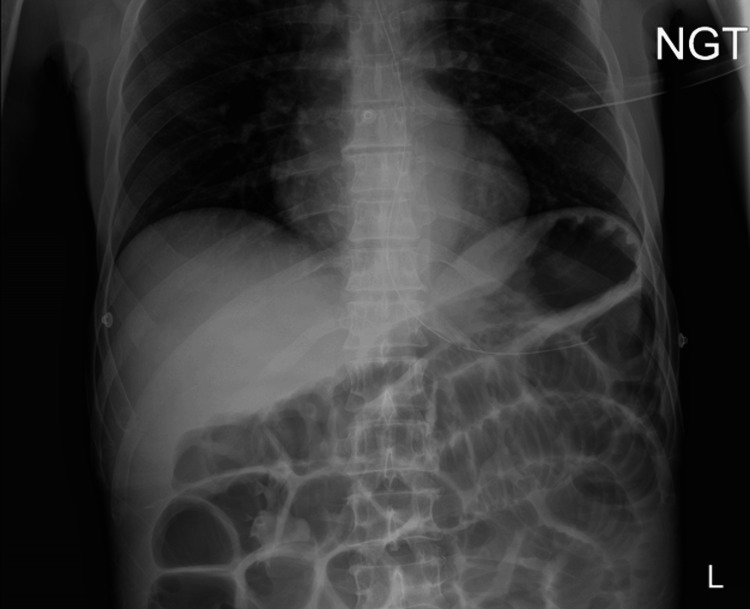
X-ray of the abdomen. The image depicts diffuse elevated loops of the small bowel with a nasogastric tube (NGT) in place.
